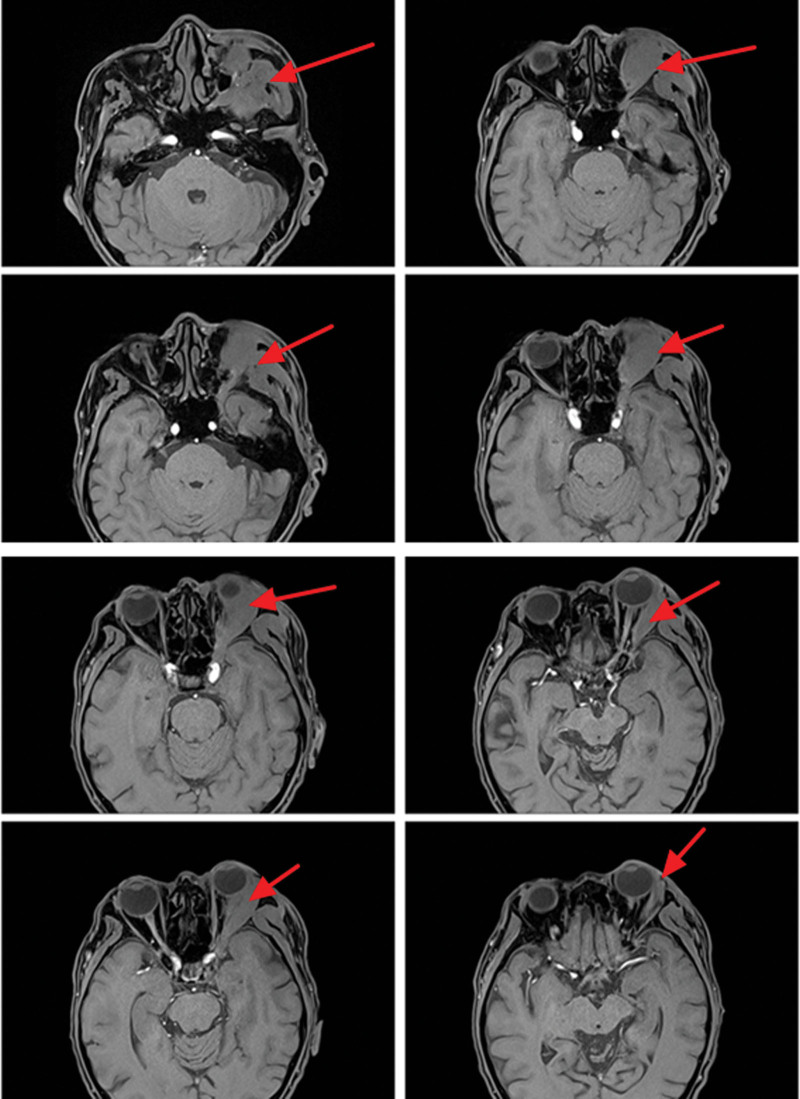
Patient MRI cross-sectional T1 phase with isosignal nonsignificant enhancement of the left posterior fat space, left lateral eye, and left inferior muscle space masses (red arrows). MRI = magnetic resonance imaging.
